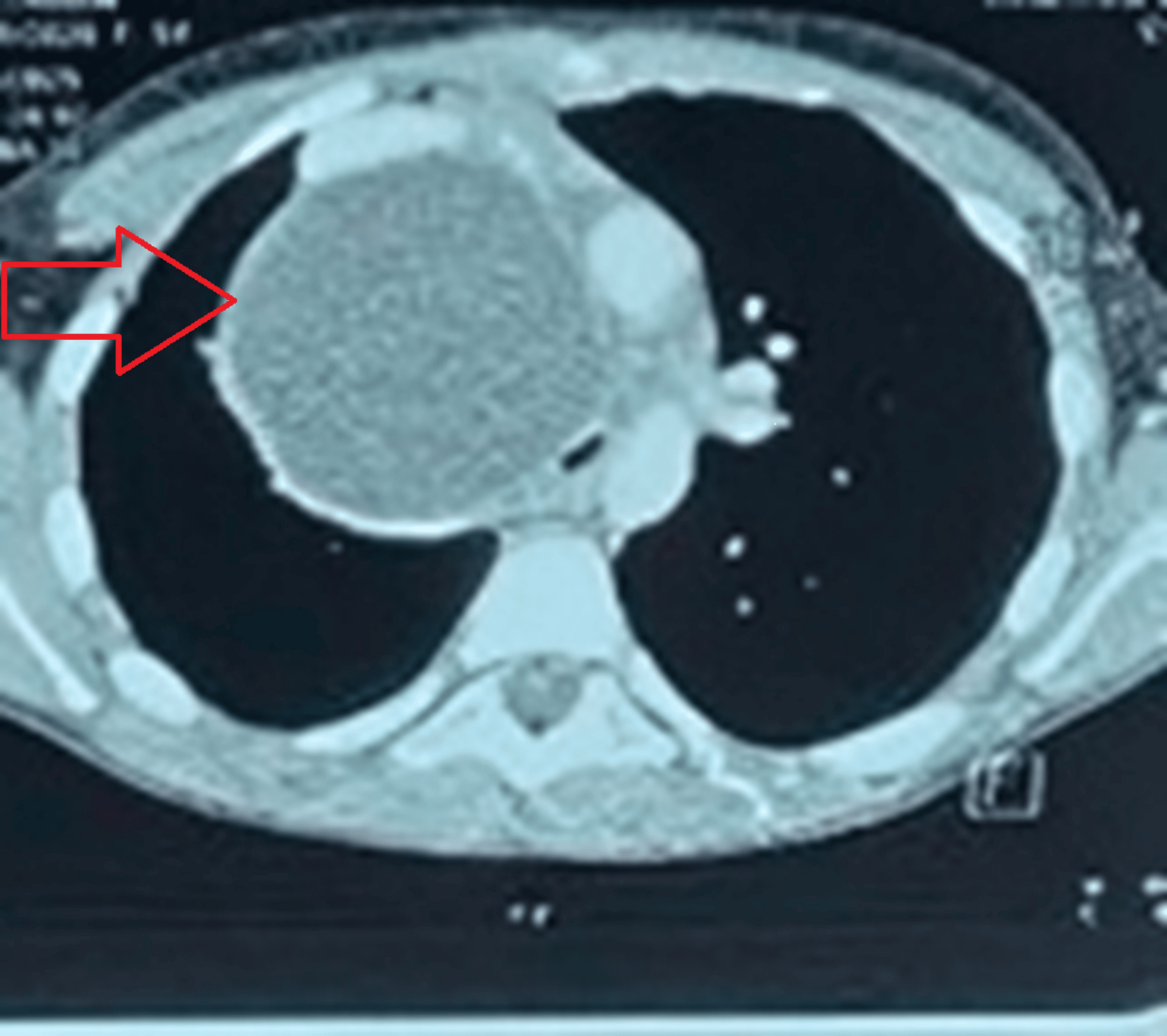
Chest computed tomography scan showing an isolated cystic lesion in the middle mediastinum (red arrow), compressing the trachea and the superior vena cava.
